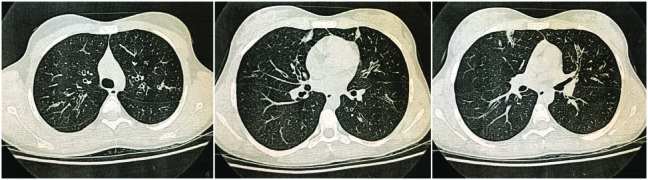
High-resolution computed tomography of the thorax showing ectatic bronchi. Thickened bronchial wall, with an impaction inside the lumen in both upper lung lobes, the middle lobe, and the lingula, is visible. Lymphadenopathy or pleural effusion is not visible.
